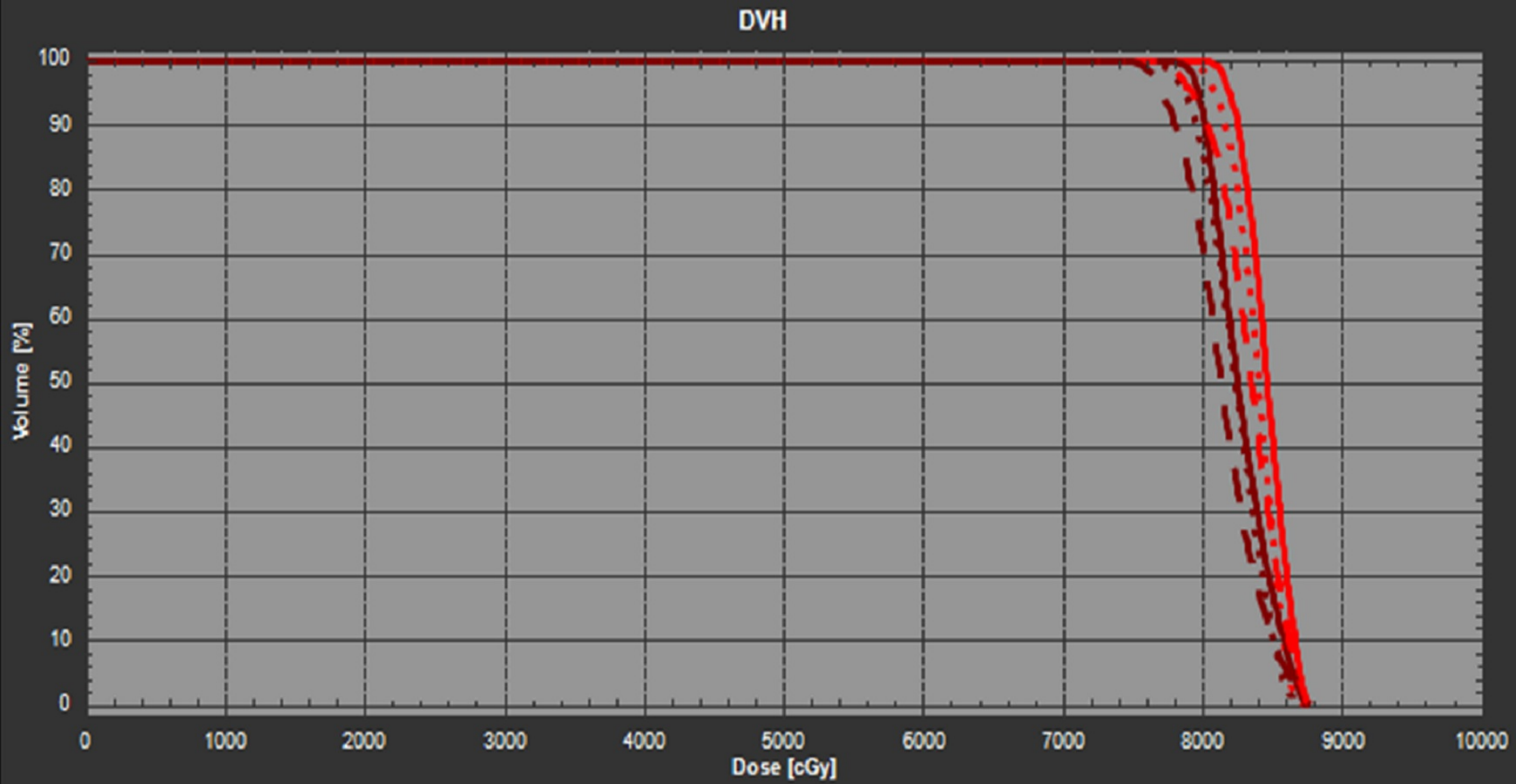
The DVH of the RR plan in the planning CT (solid line), +3 degree (CW) rotated CT (dashed line), and -3 degree (CCW) rotated CT (dotted line). The red lines denote the iGTV and the dark brown lines denote the CTV. CCW: Counter-clockwise; CT: Computed tomography; CTV: Clinical target volume; CW: Clockwise; DVH: Dose volume histogram; iGTV: internal gross target volume; RR: Roll robust.
